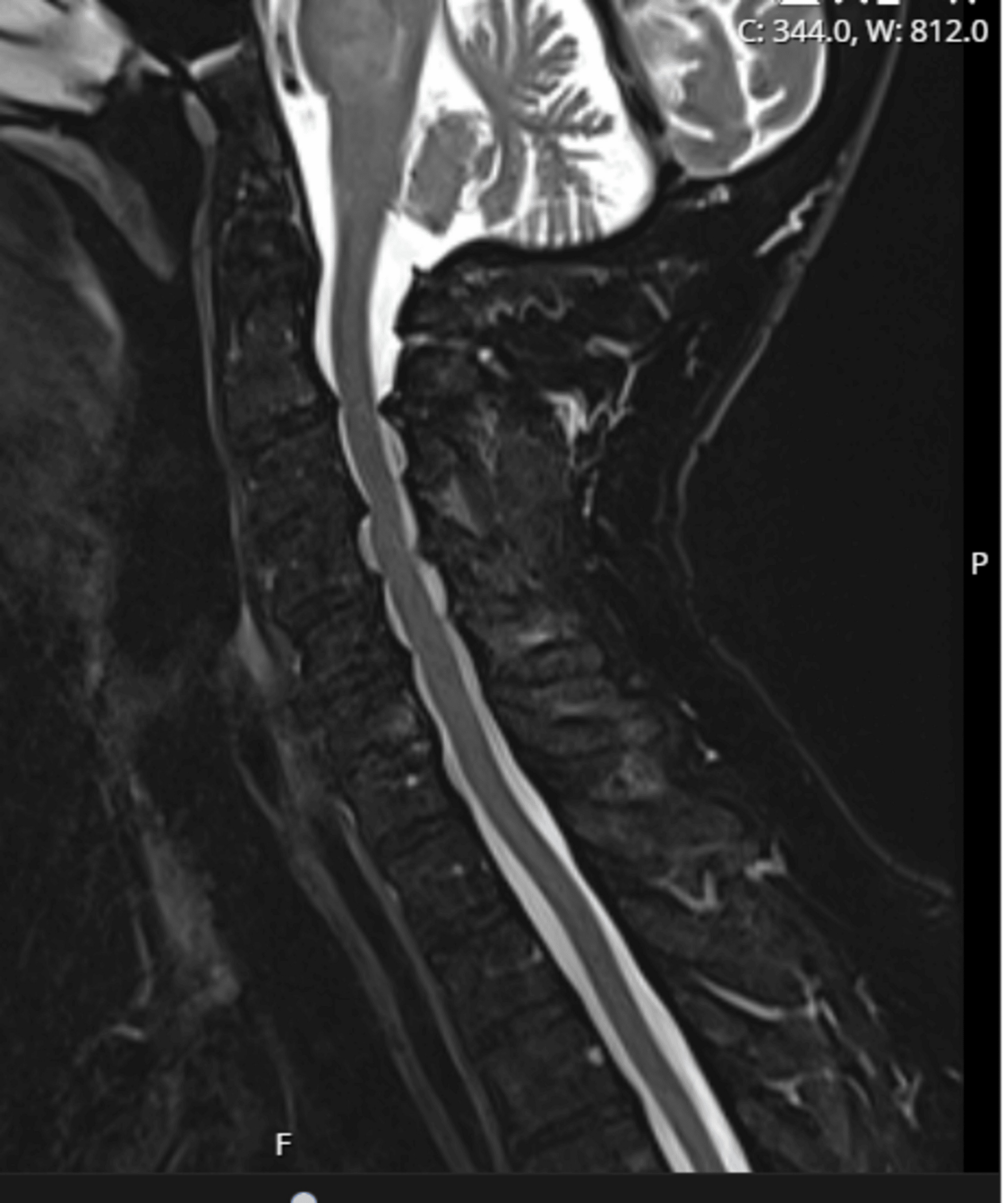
Sagittal T2 weighted MRI of the brainstem and cervical spine Sagittal T2-weighted MRI of the brainstem and cervical spine shows normal anatomy.
